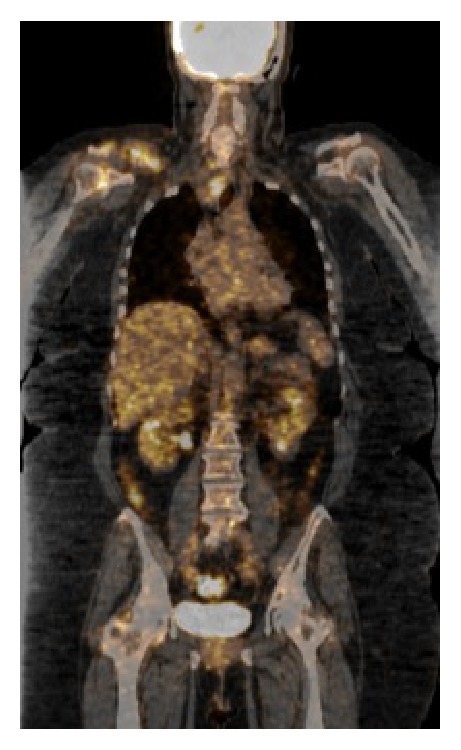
Coronal-fused 18F-FDG PET/CT shows FDG avid lesion in the distal right clavicle with osseous destruction and an FDG avid mediastinal mass displacing the trachea to the left.
